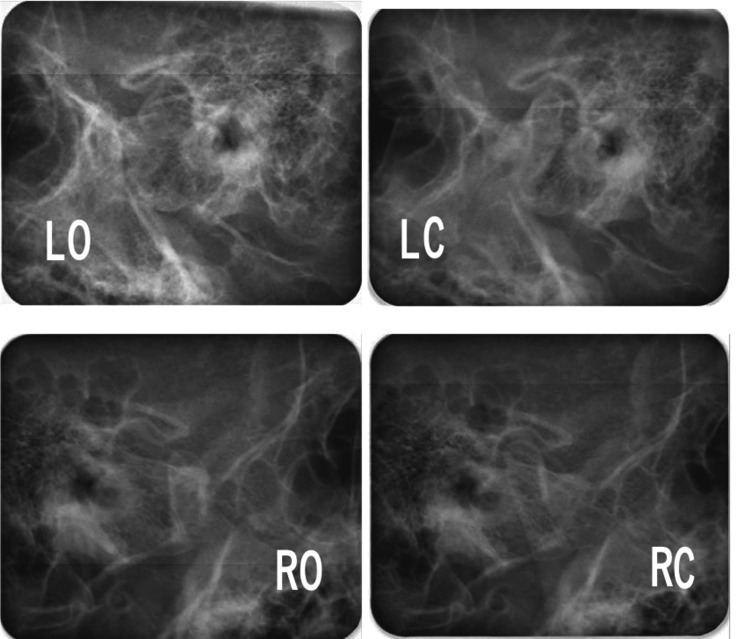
Post-treatment evaluation. Schuller’s Radiograph. LO, left open; LC, left close; RO, right open; RC, right close.
